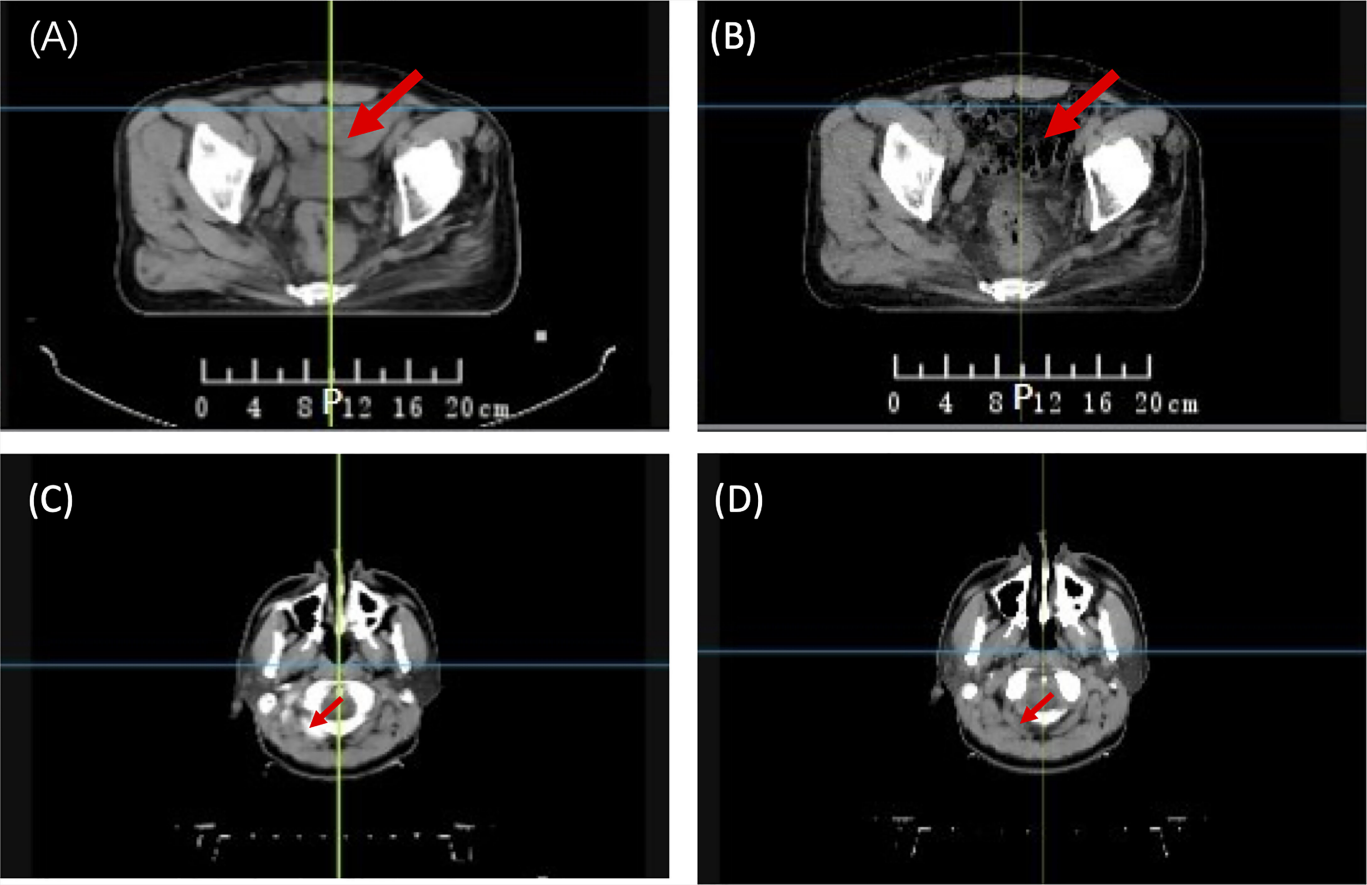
Anatomical variations between planning CT (pCT) and daily CT (dCT). (A) pCT of a rectal patient; (B) dCT of a rectal patient; (C) pCT of an NPC patient; and (D) dCT of an NPC patient. NPC, Nasopharyngeal Carcinoma.
